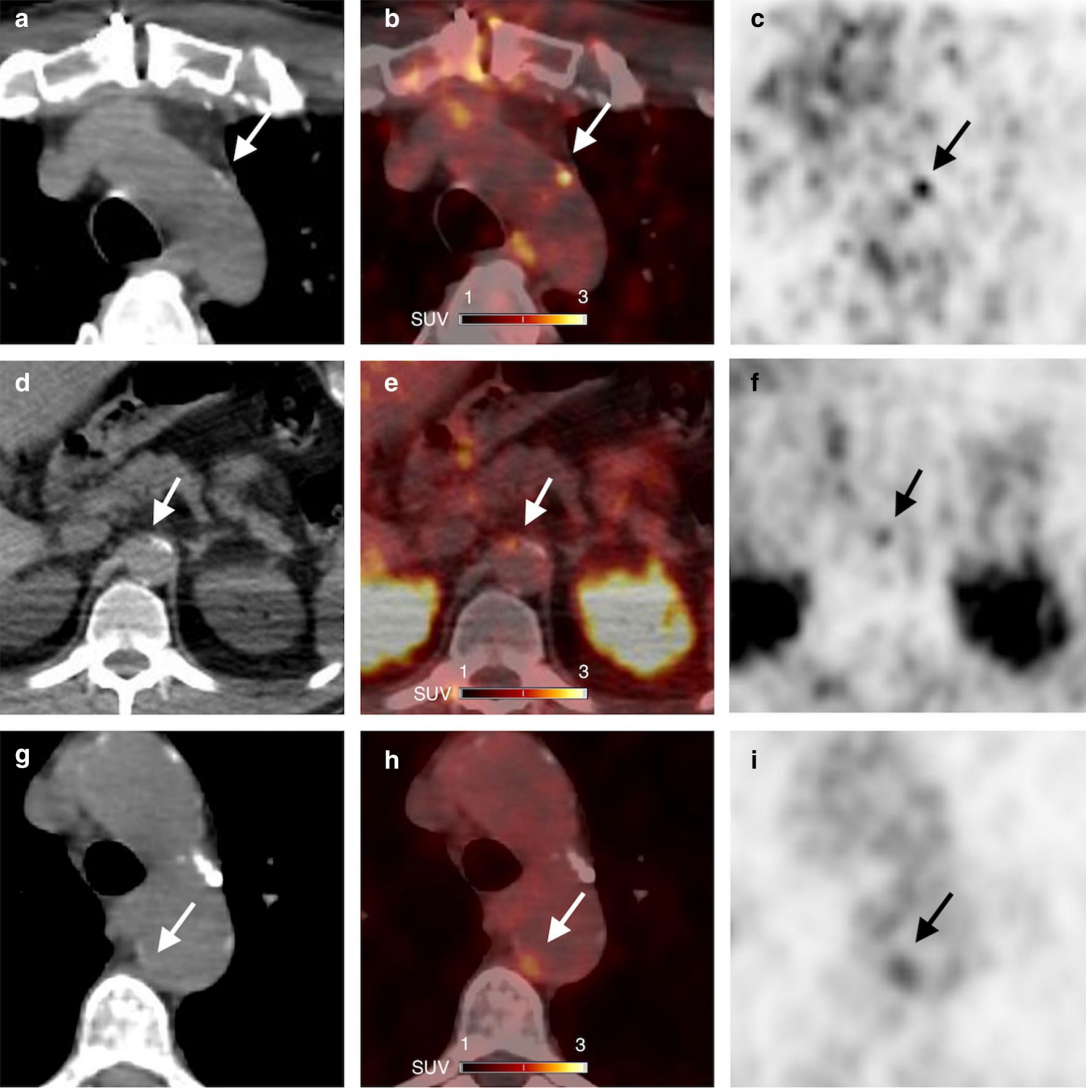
CT (A, D, G), PET/CT (B, E, H) and PET images (C, F, I) in axial views show foci of ^68^Ga-NODAGA-RGD arterial uptake in partly calcified atherosclerotic lesions (arrows): (A, B, C) in wall of arch aorta in a 62-year-old man who had a myocardial infarction 5 months before PET imaging; (D, E, F) in wall of abdominal aorta in a 54-year-old man who had a transient ischemic attack one week before PET imaging; (G, H, I) in wall of arch aorta in a 74-year-old woman who had a myocardial infarction 33 months before PET imaging
